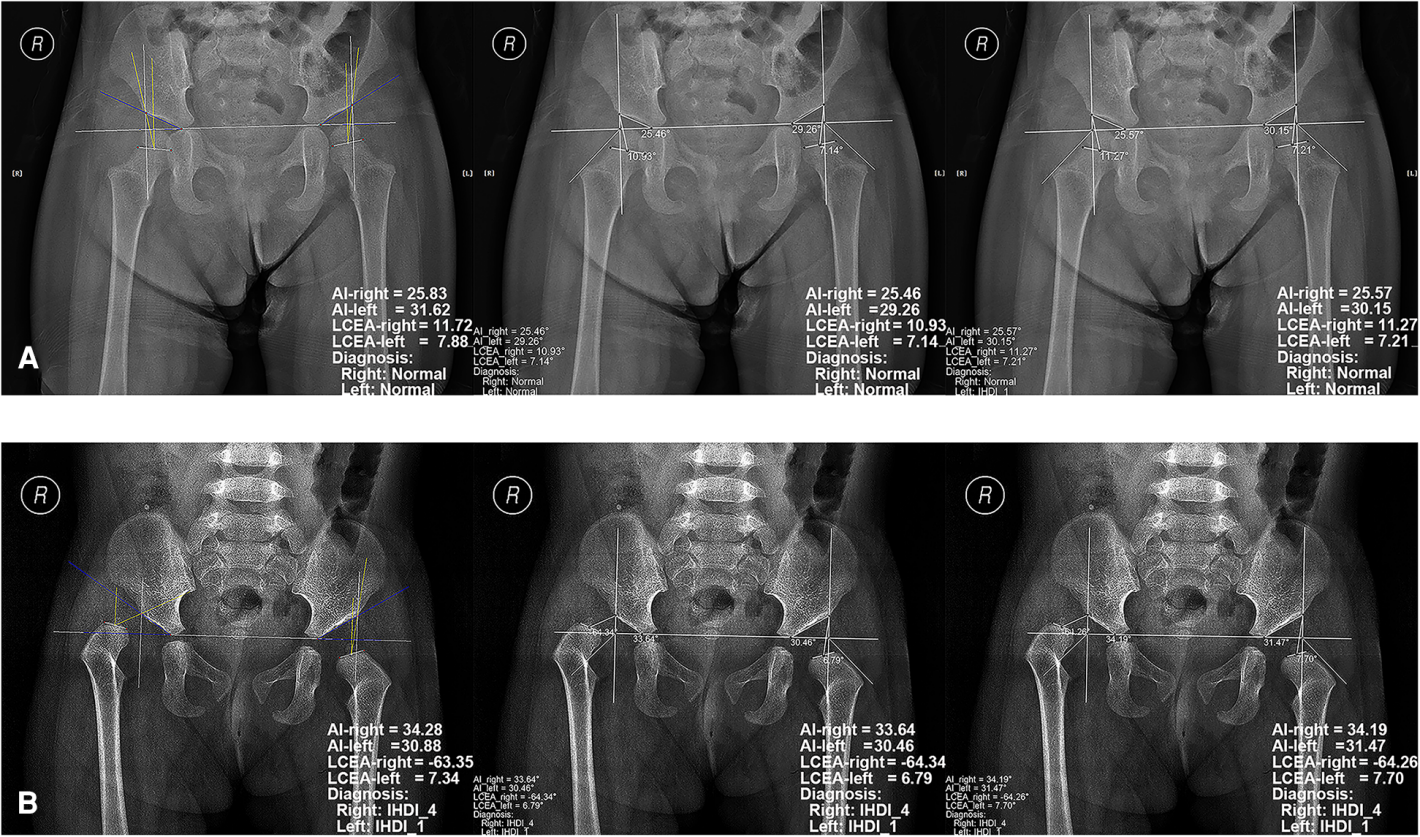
Examples of radiographic diagnosis of developmental dysplasia of the hip on pelvic radiographs by the software. The left images represent the evaluation results of the standard, while the middle and right images represent those of the software by Junior orthopedist and Intermediate orthopedist, respectively. (A) the pelvic radiograph of a 2.3-year-old girl; (B) the pelvic radiograph of a 1.4-year-old girl.
